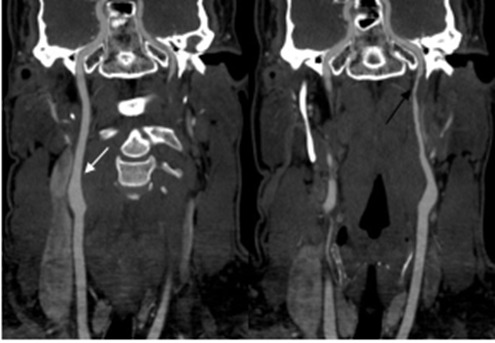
Coronal computed tomography demonstrating patent right internal carotid artery (white arrow) and the stenosis of the left internal carotid artery depicting the “carotid string sign” (black arrow).
